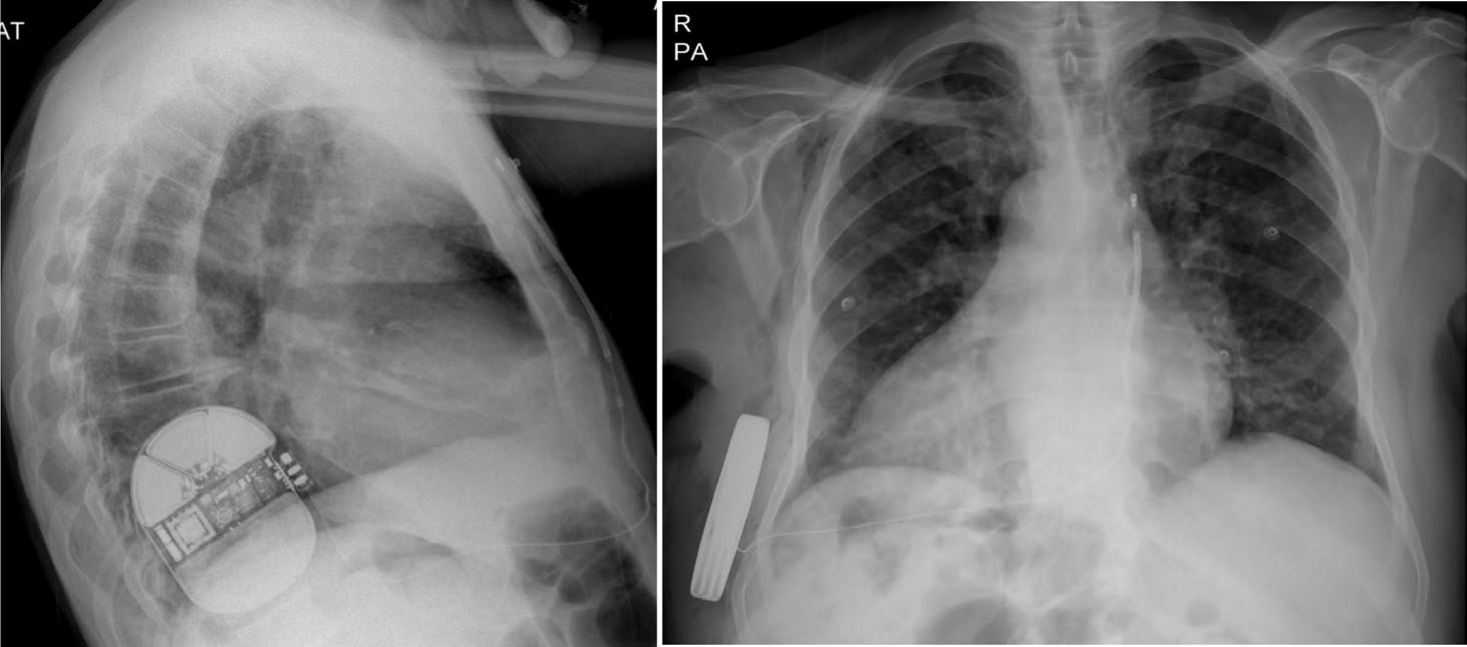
Posteroanterior and lateral chest X‐rays after implantation, confirming appropriate positioning of the S‐ICD generator in the right midaxillary line and the defibrillation electrode along the left parasternal area.
